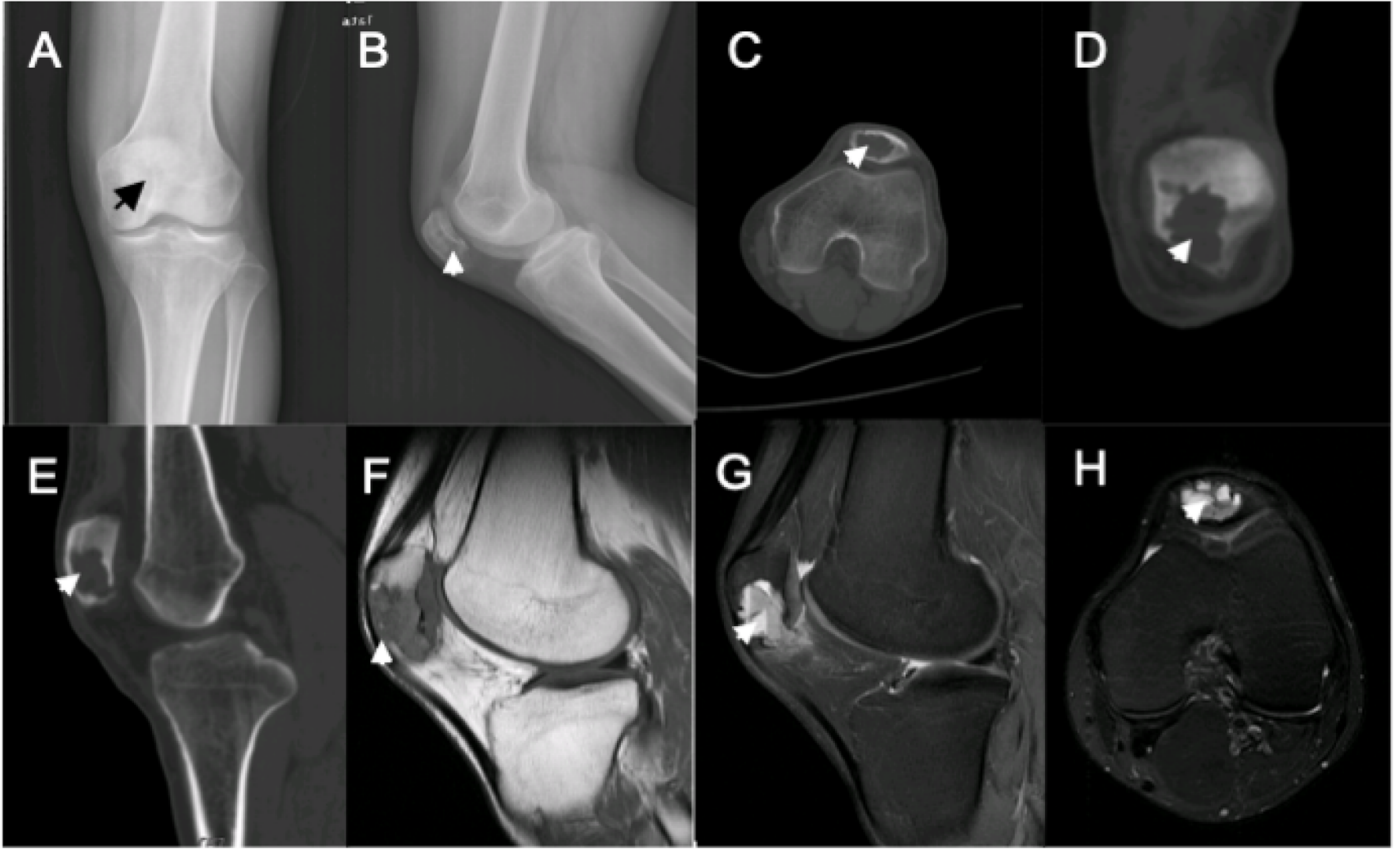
Preoperative imaging results (a, b). The radioparent lesion in the patella can be seen in the anteroposterior and lateral X-rays (c, d). The focus with a sharp edge, in a lobulated or worm-eaten shape, was observed in the axial and coronal views of preoperative CT. No boundary or internal calcification was noted in the focus (e). At the sagittal view of preoperative CT, the focus was connected with the joint cavity at the junction between the inferior patella and the articular surface (f-h). In preoperative MRI, f was T1WI, the patella focus was hypointensity, and g and h were T2WI. The focus was hyperintense and contained the fluid level. Therefore, secondary ABC was suggestive
